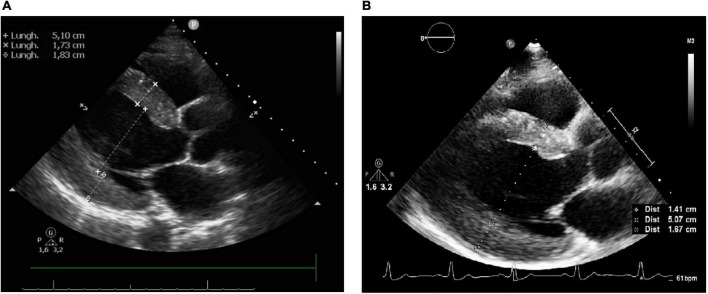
(A) Echocardiography after 2 months since the admission. (B) Echocardiography after 4 months since the admission.
